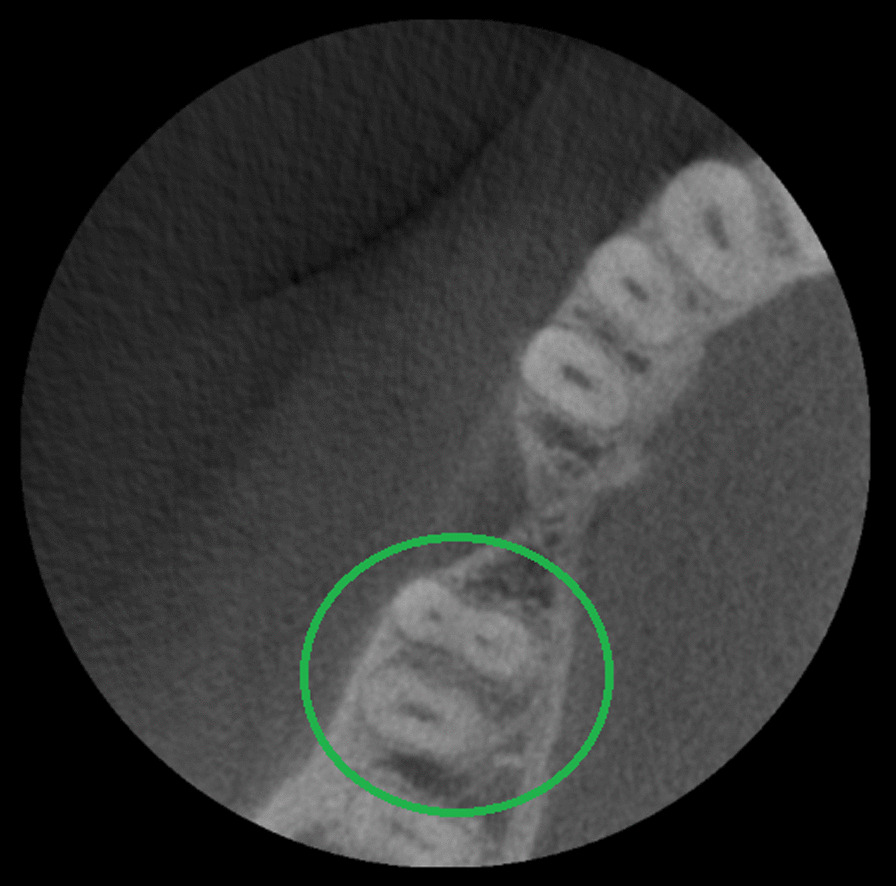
Axial view from CBCT scan showing mandibular second molar with two roots
